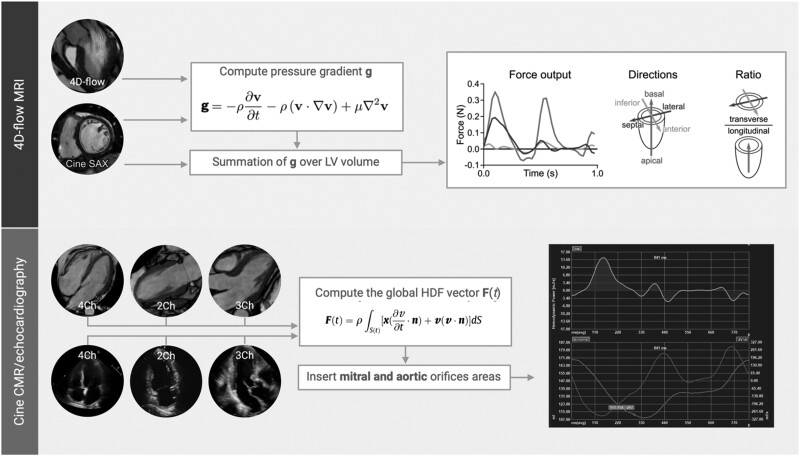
Techniques currently used to assess haemodynamic forces (HDFs). Upper panel: Global haemodynamic forces (HDFs) can be calculated at 4D-flow magnetic resonance imaging (MRI). Left ventricular (LV) endocardial border is manually delineated in cine images. Once the velocity field is known, pressure gradients g in the 4D-data set are computed using the Navier–Stokes equation and integrated over the LV ventricular volume to produce the HDF. The equation uses variables such as *ρ* for mass density, *v* for fluid velocity vector field, *t* for time, and *μ* for the fluid viscosity. The force vector obtained is decomposed into 3 orthogonal components: basal–apical, lateral–septal, and inferior–anterior. For each component, HDF output, directions, and the transverse/longitudinal ratio are obtained. Lower panel: Thanks to recent advances,^1^ HDFs can be also computer at cine cardiac magnetic resonance (CMR) and echocardiographic images. First, a four, two, and three-chamber view should be acquired. The global HDF vector F(t) is then calculated thanks to the equation here reported. The fluid velocity vector field *v*(*x, t*) is measured at fixed points *x* at time *t*, while *S*(*t*) represents the closed surface bounding the volume, and *n* is the outward unit normal vector. In this way, the force associated with a fluid volume can be evaluated from measurements carried out at the boundaries of the volume regardless of flow phenomena developing inside. This calculation, in fact, requires the velocity over the endocardial boundary (derived from the myocardial movement using the same feature tracking results used for strain and strain rate analysis) and the blood velocity across the valves (which is calculated from the volumetric changes of the LV and the valve area, using the conservation of mass principle). The three-dimensional surface is reconstructed by combining the 3 apical views, and parameters similar to 4D flow are obtained (i.e. haemodynamic force, orientation, and angle ratio). For an explanation of the individual curves shown in the last part of the lower panel, please refer to *Figure 3*.
